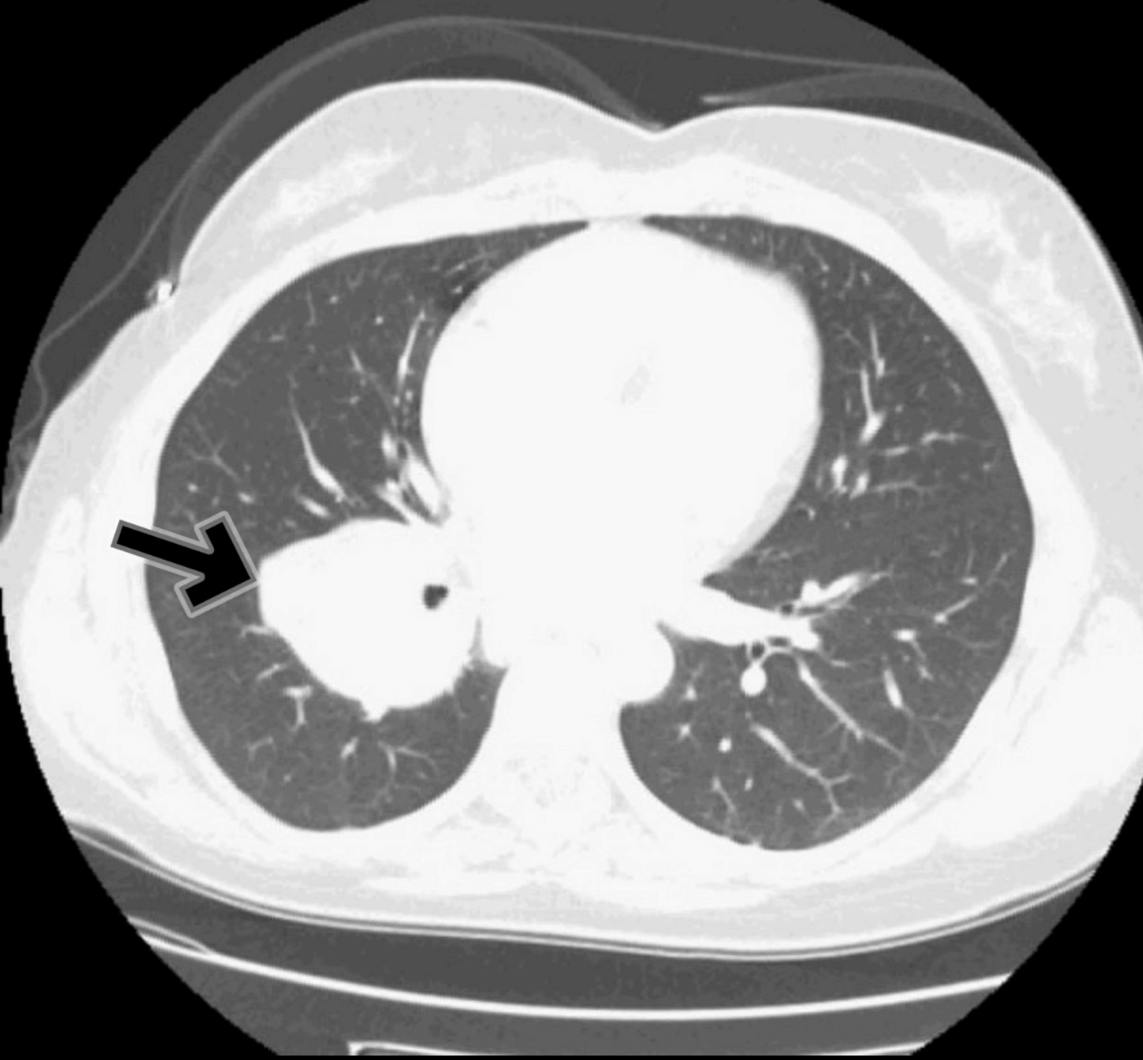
CT scan of the thorax Confirmed right perihilar mass (arrow)
